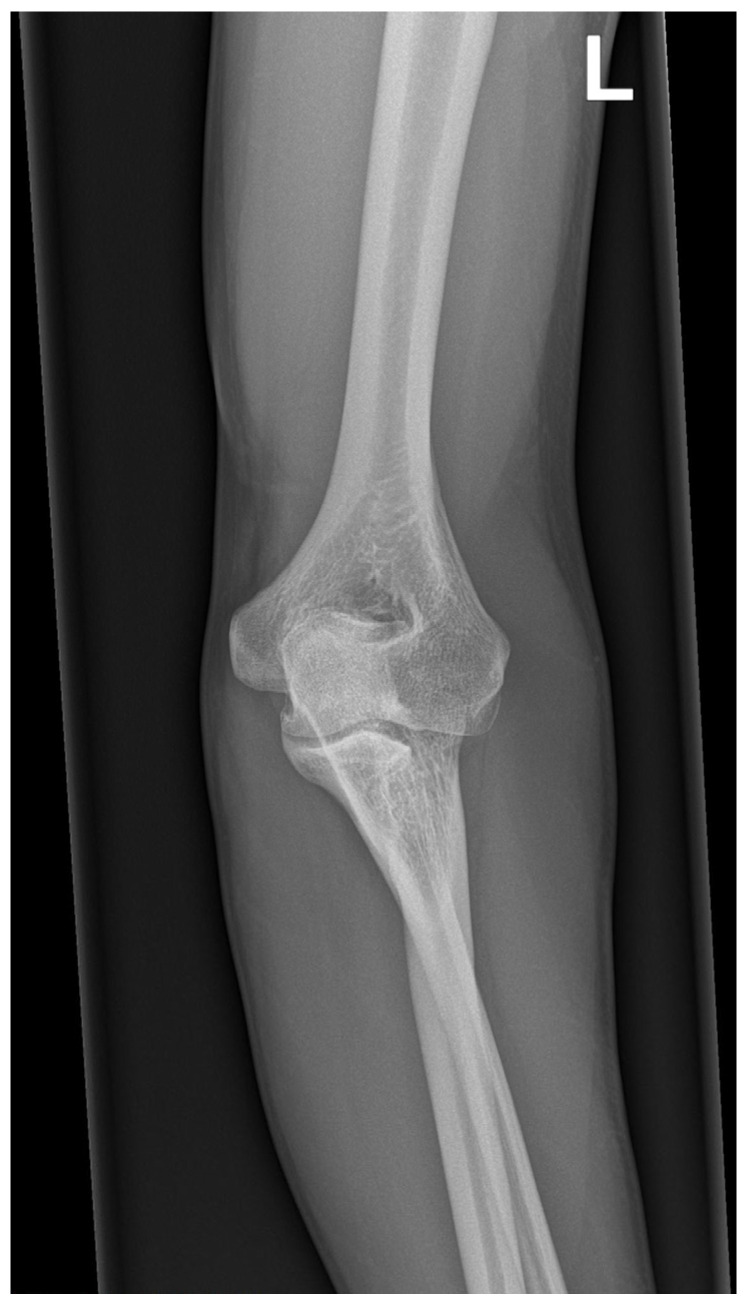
Radiographic image of the elbow joint in antero-posterior view showing Cleary–Omer Type III PRUS.
